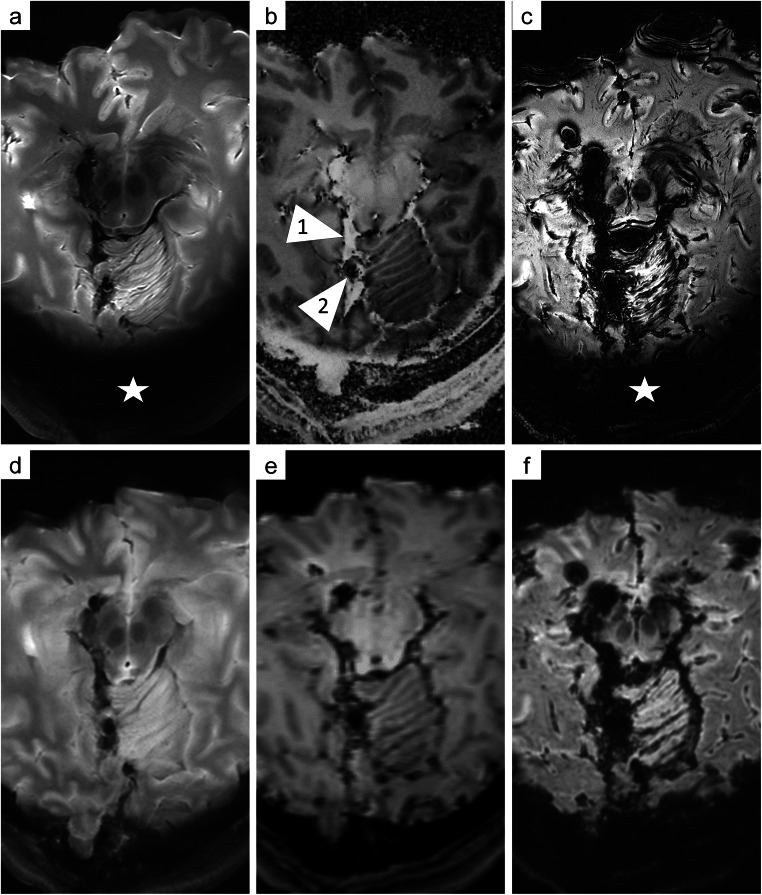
Comparison of 7 T images (a: T_2_-SPACE; b: MP2RAGE; c: SWI) and 3 T images (d: T_2_-TSE-SPAIR; e: T_1_-TFE; f: VenBold) obtained in case 1 and aligned to the bullet path. The wound tract and soft tissue injuries along the bullet path, such as the injury to the pedunculus cerebri, were delineated with high resolution on 7 T MRI but were blurry on 3 T images. The T_2_-SPACE sequence (a) demonstrated higher spatial resolution and soft tissue contrast, which allowed a detailed depiction of the wound tract to be obtained. The MP2RAGE sequence (b) allowed clear distinction between blood (arrowhead 1: hyper intense, bright) and gas (arrowhead 2: hypo intense, dark) and additionally provided high contrast between gray and white matter. Although the SWI sequence (c) presented very high spatial resolution, the radiologic assessment was severely impeded by intracranial gas cavities. Signal dropout was observed in the lower occipital region (asterisk)
